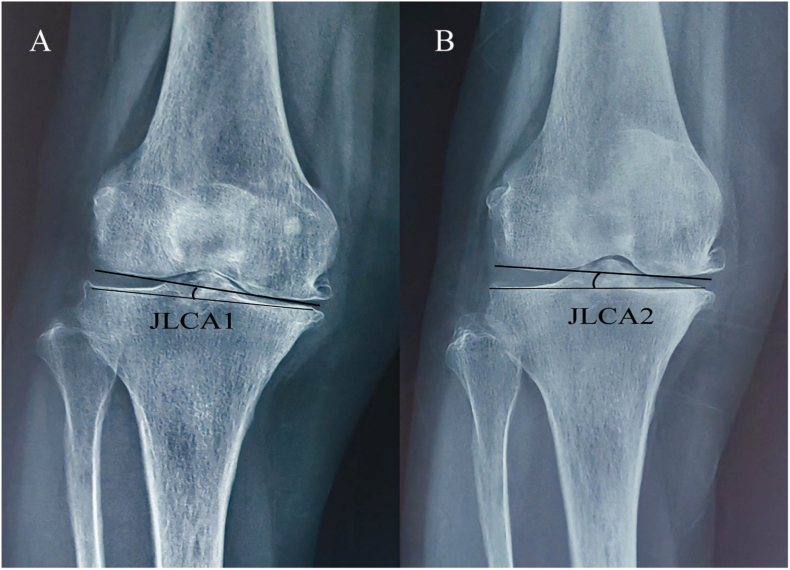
The disparity in JLCA between the supine (JLCA2) and upright (JLCA1) X-ray films is denoted as ΔJLCA; Joint line convergence angle (JLCA).
